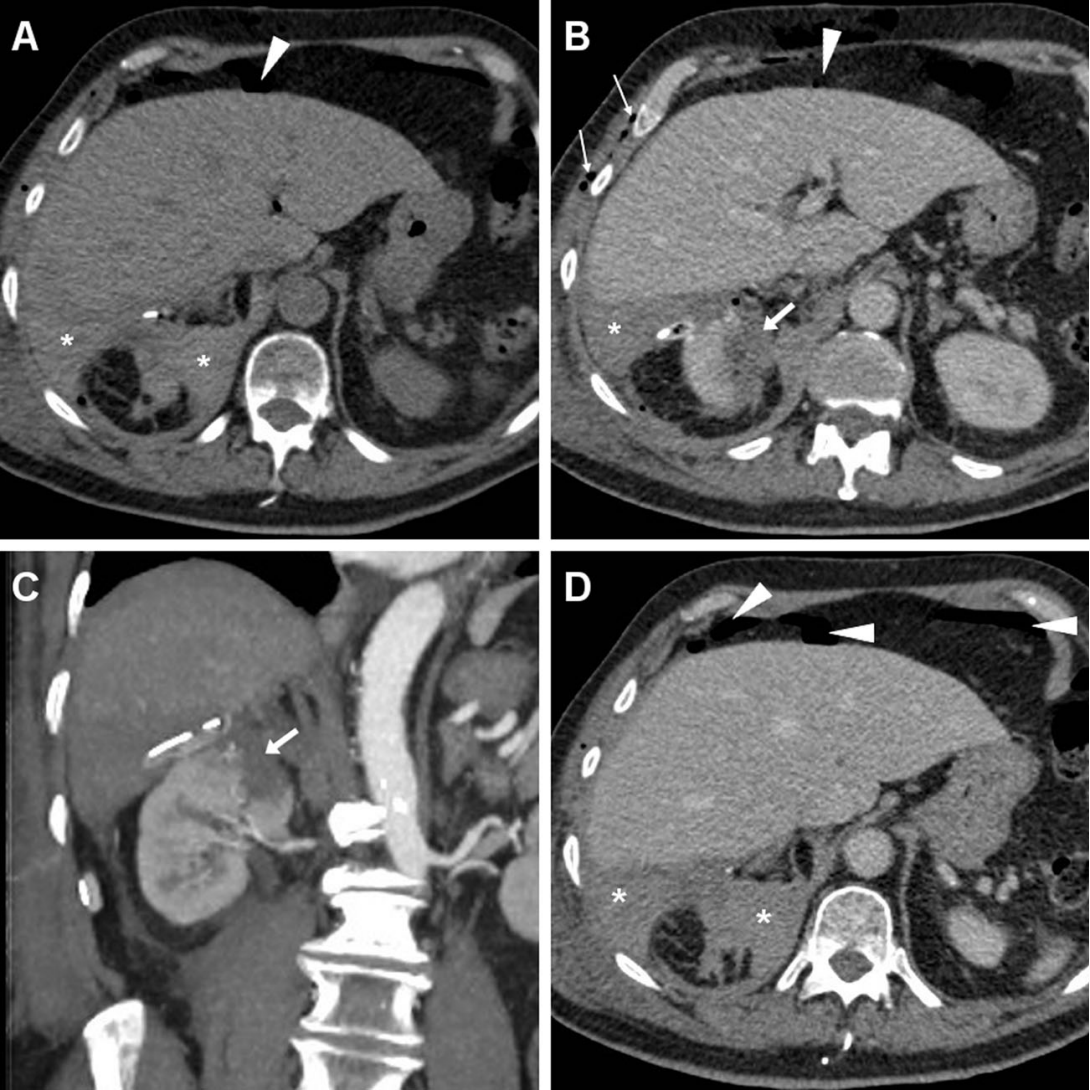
Haematoma in a 74-year-old man with diabetes, cardiac pacemaker, and progressive postoperative blood loss after L-PN. Unenhanced (a) and post-contrast (b, c) Multidetector CT images showed residual intraperitoneal gas bubbles (*arrowheads*) and minimal emphysema of the abdominal wall (*thin arrows* in b). Hyperattenuating blood collection (*) with the distal end of the drainage tube was seen along the posterior aspect of the right liver lobe, abutting the upper right renal pole with the hypoenhancing resection site (*arrows* in b, c) without CM extravasation indicating active bleeding in the corticomedullary phase (b, c) or urine leak in the excretory phase (d). The patient recovered with transfusion support
